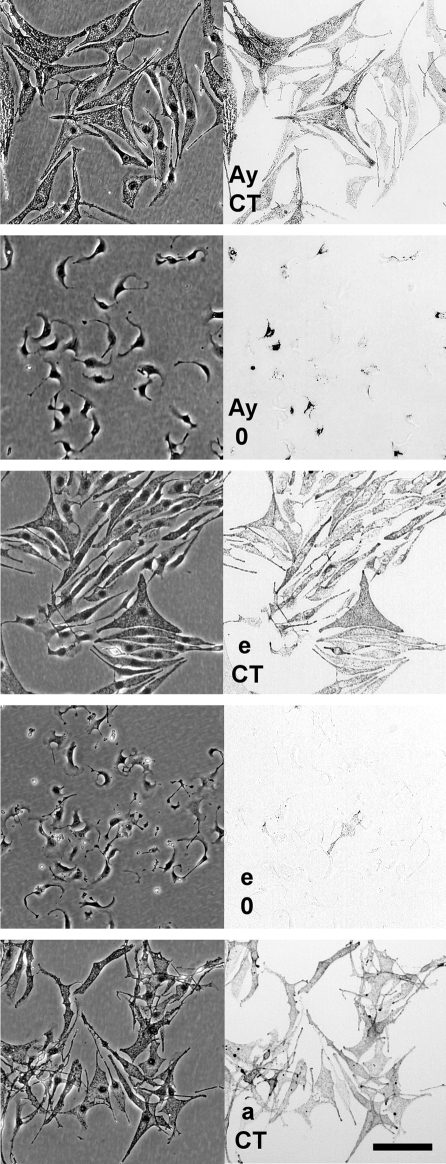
Melanoblast-like appearance of melan-Ay and melan-e cells in the absence of CT. Paired phase-contrast (left) and bright-field (right) images of melan-Ay (Ay) and melan-e (e) cells grown with 20 pM CT (CT) or for several passages without CT (0). Some residual eumelanin is seen in some cells of both genotypes without CT. For reference melan-a cells (a) grown with 20 pM CT are also shown; there is little difference from melan-a cells without CT (Figure 1). Bar = 100 μm.
